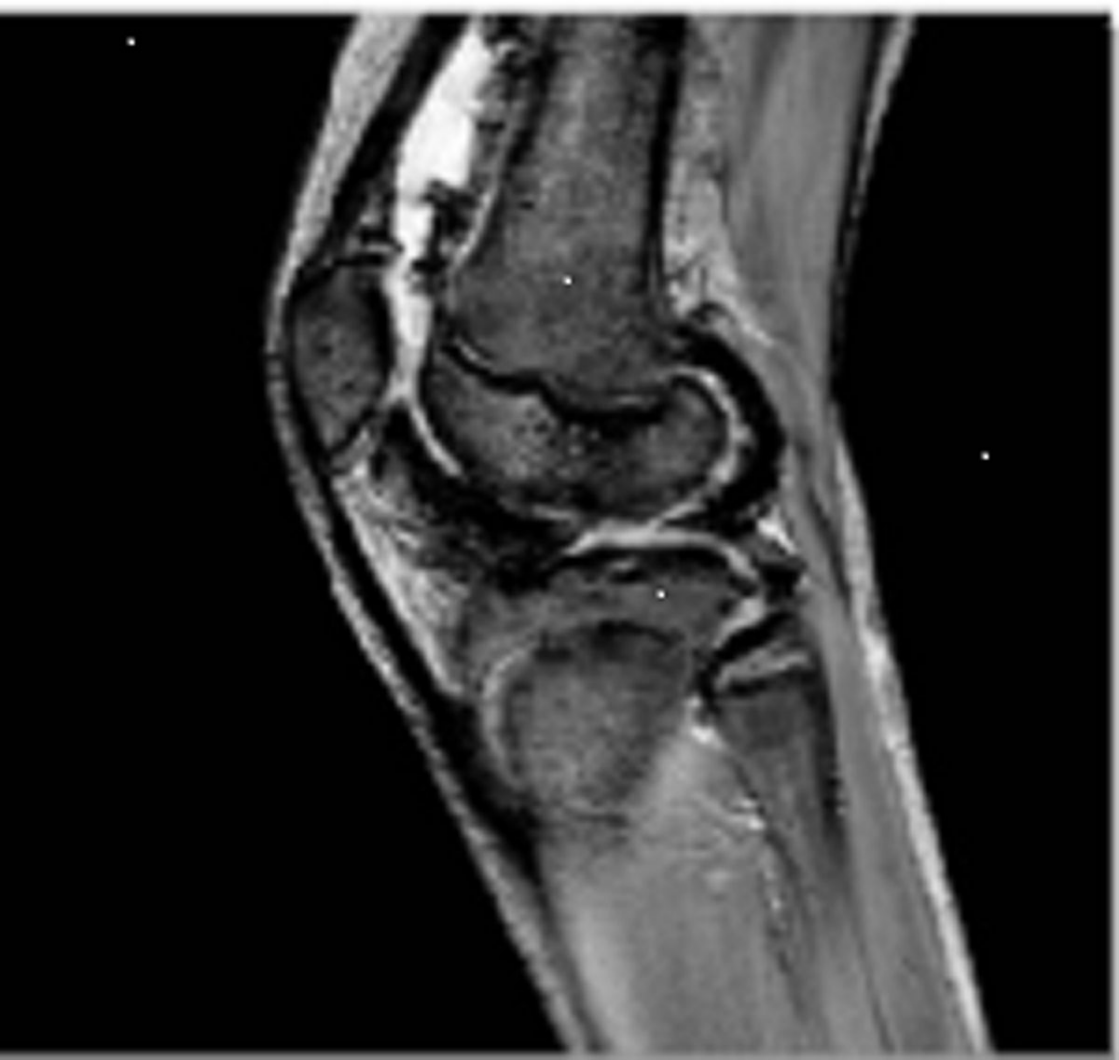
Gradient echo MRI (2012) Haemosiderin deposition noted
